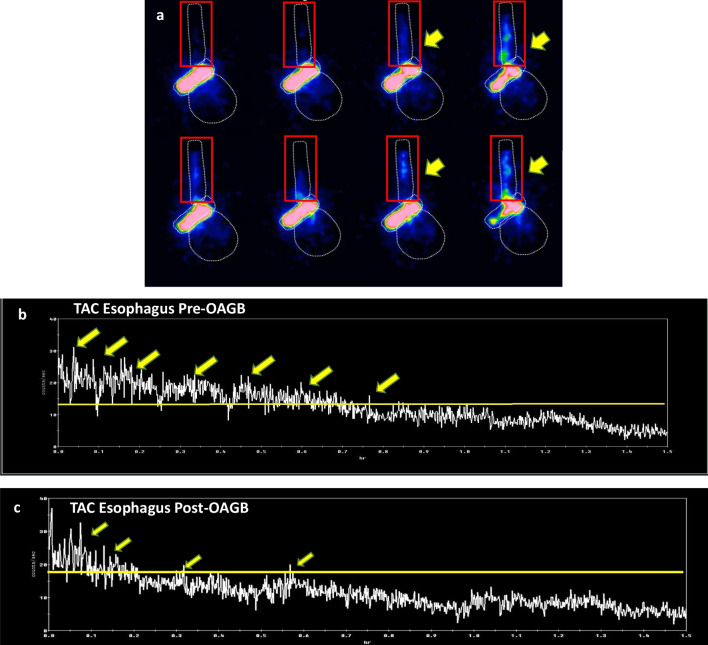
Nuclear scintigraphy post-prandial reflux. a Serial images of post-prandial reflux seen on a high-resolution gastric emptying study, using semi-solid bolus at a rate of 5 s per frame. The ROI were drawn around the esophagus, stomach, and small bowel. Reflux into the mid-esophagus and upper esophagus was measured with an ROI depicted by the red solid line. Reflux events were depicted by the yellow arrows. b toc Time-activity curve of the upper and mid-esophagus displaying reflux events (yellow arrows). The yellow horizontal line indicates the minimal threshold of reflux
